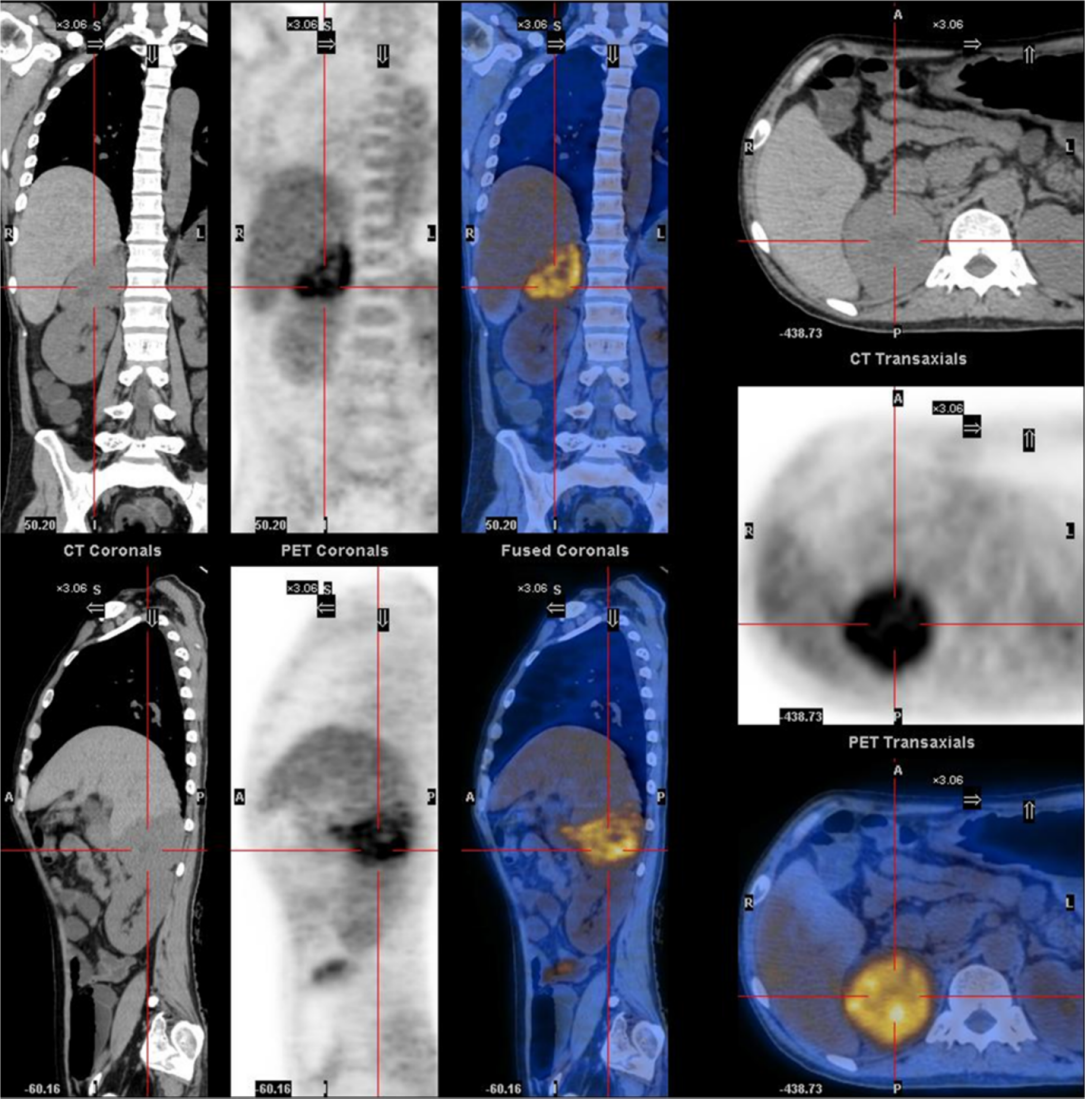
A PET-CT scan demonstrating a large, well defined adrenal mass. A large hyper-metabolic mass was located at the right adrenal region. The hypodense areas at the center indicated necrosis. Massive fluid collection could be found in the colon.
